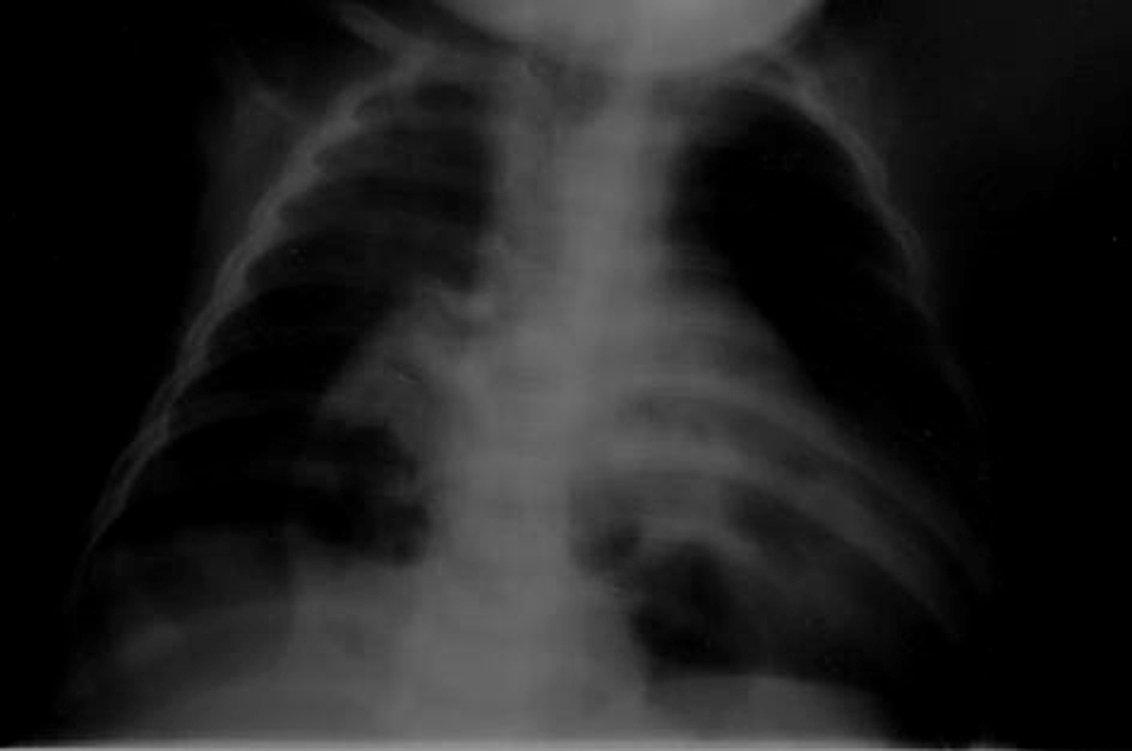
Posteroanterior chest X-ray. Bowel loops compressing the right ventricle of the heart.
